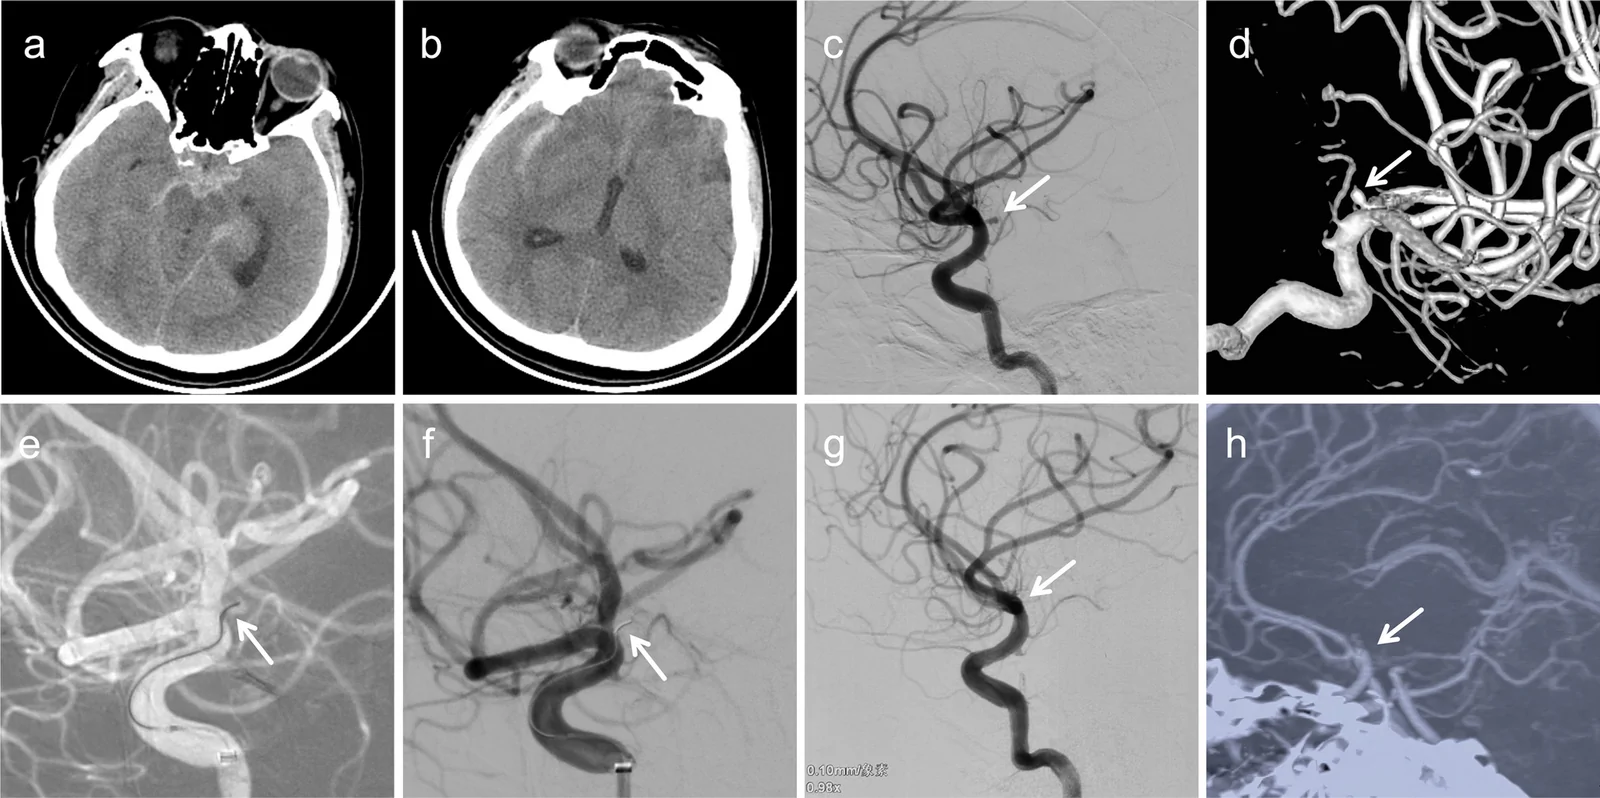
a, b Cranial CT showed the presence of a subarachnoid hemorrhage. c, d Lateral angiography and 3D-RA demonstrated a tiny aneurysm (arrow) arising from the A1 segment of the right anterior cerebral artery. The aneurysm exhibited a blister-like appearance with a narrow neck (arrow). e The tip of the Traxcess-14 guidewire was positioned within the aneurysm cavity, and the microcatheter was advanced close to the neck of the aneurysm (arrow). f After 5 cycles of electrocoagulation, the aneurysm opacification disappeared (arrow). g Lateral angiography repeated 20 min later demonstrated complete aneurysm occlusion. h Cranial CTA performed 2 days after procedure showed no opacification of the aneurysm
